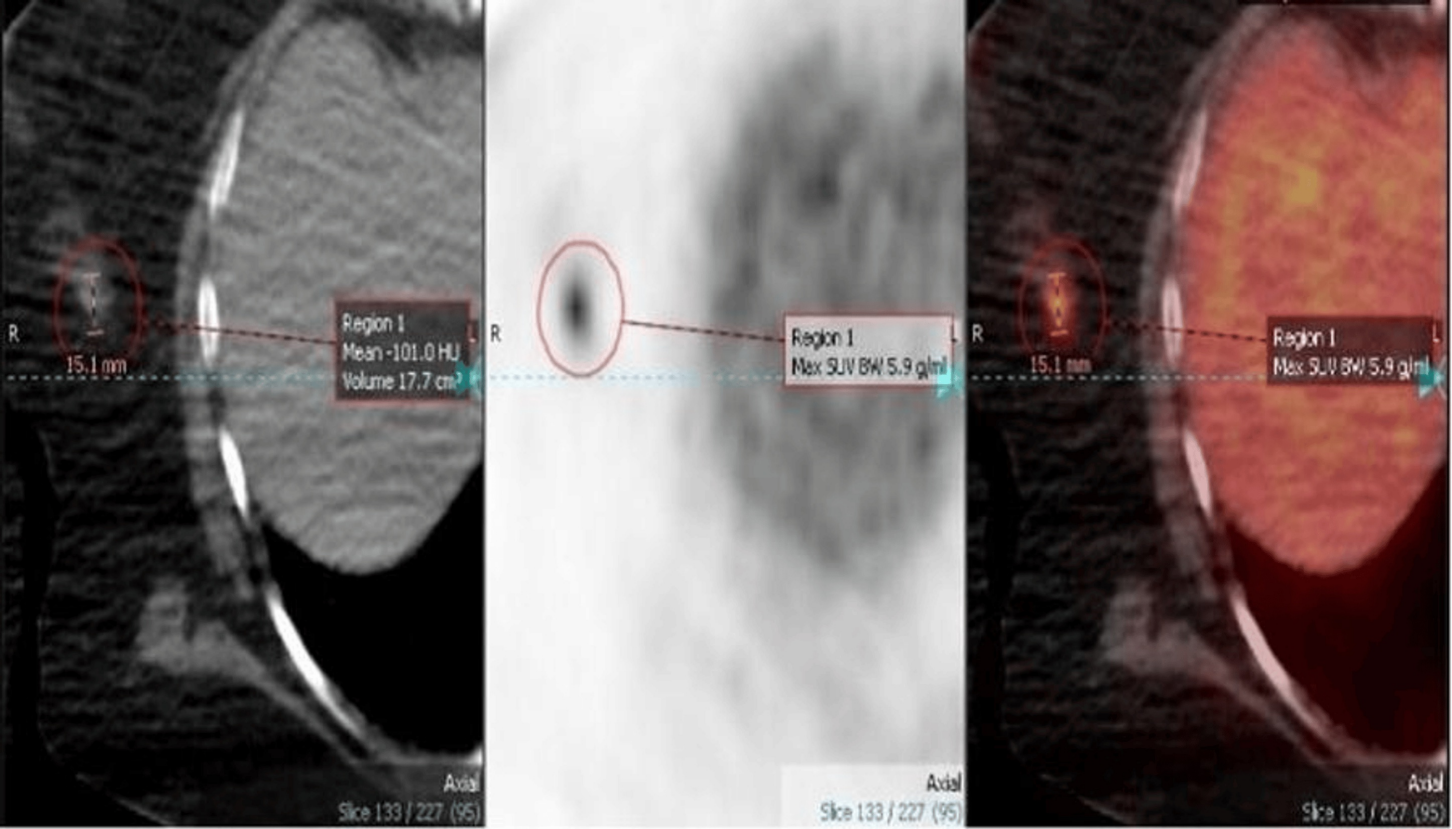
Whole-body FDG PET-CT showed an intense focal radiotracer uptake within the right breast. FDG: fluorodeoxyglucose; PET-CT: positron emission tomography-computed tomography
